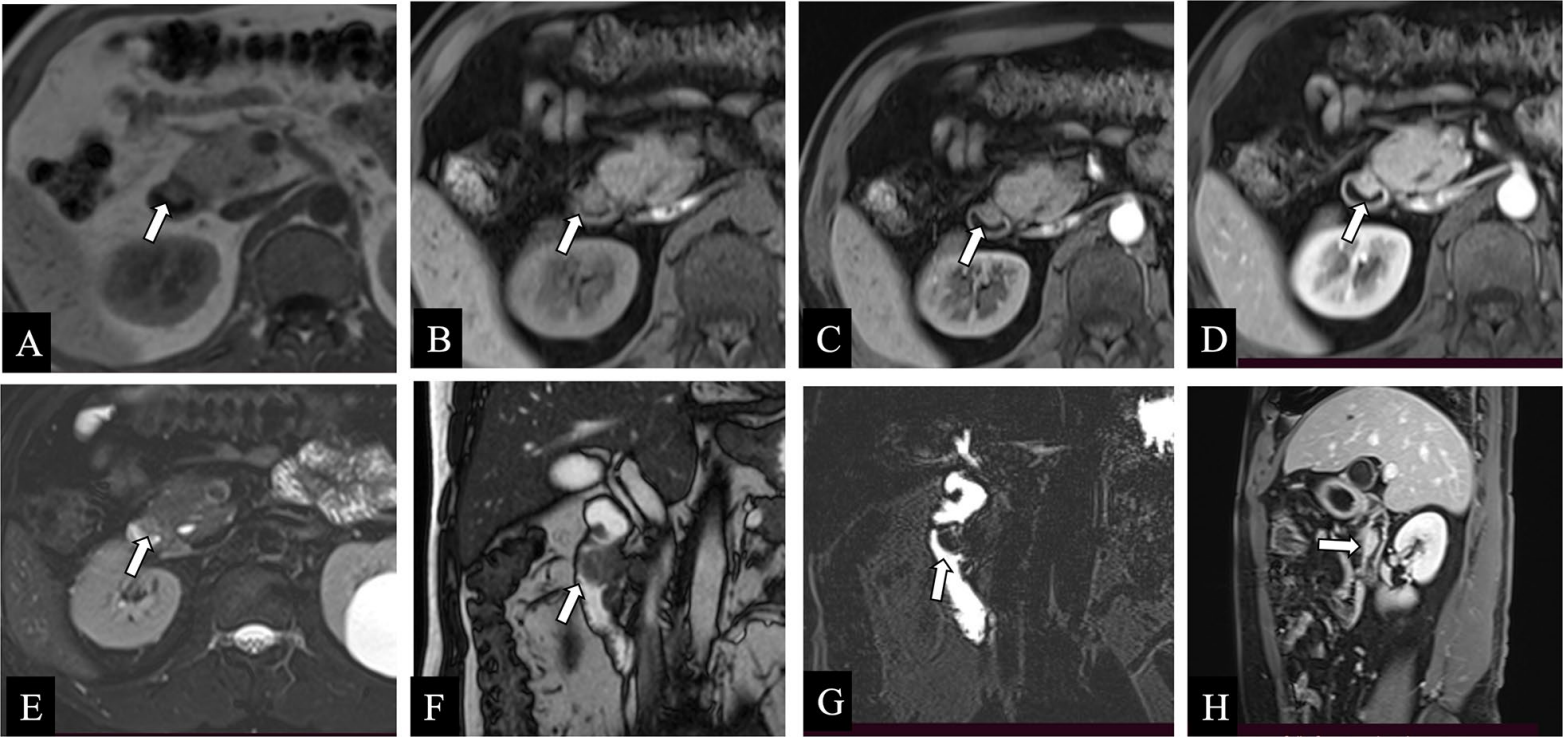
a–h Non-contrast axial T1-weighted (a), pre-contrast axial T1-weighted (b), post-contrast axial T1-weighted (c, d), and post-contrast coronal T1-weighted (h) images showed a lesion within the duodenum (white arrows) demonstrating T1-weighted hyperintense or isointense signal like those of the normal pancreas. This tissue showed similar imaging characteristics of the normal pancreas on axial T2-weighted (e) and coronal true fast image with steady-state precession (True-FISP) (f) images (white arrows). Magnetic resonance cholangiopancreatography image (g) showed a nodular filling defect in the duodenum
